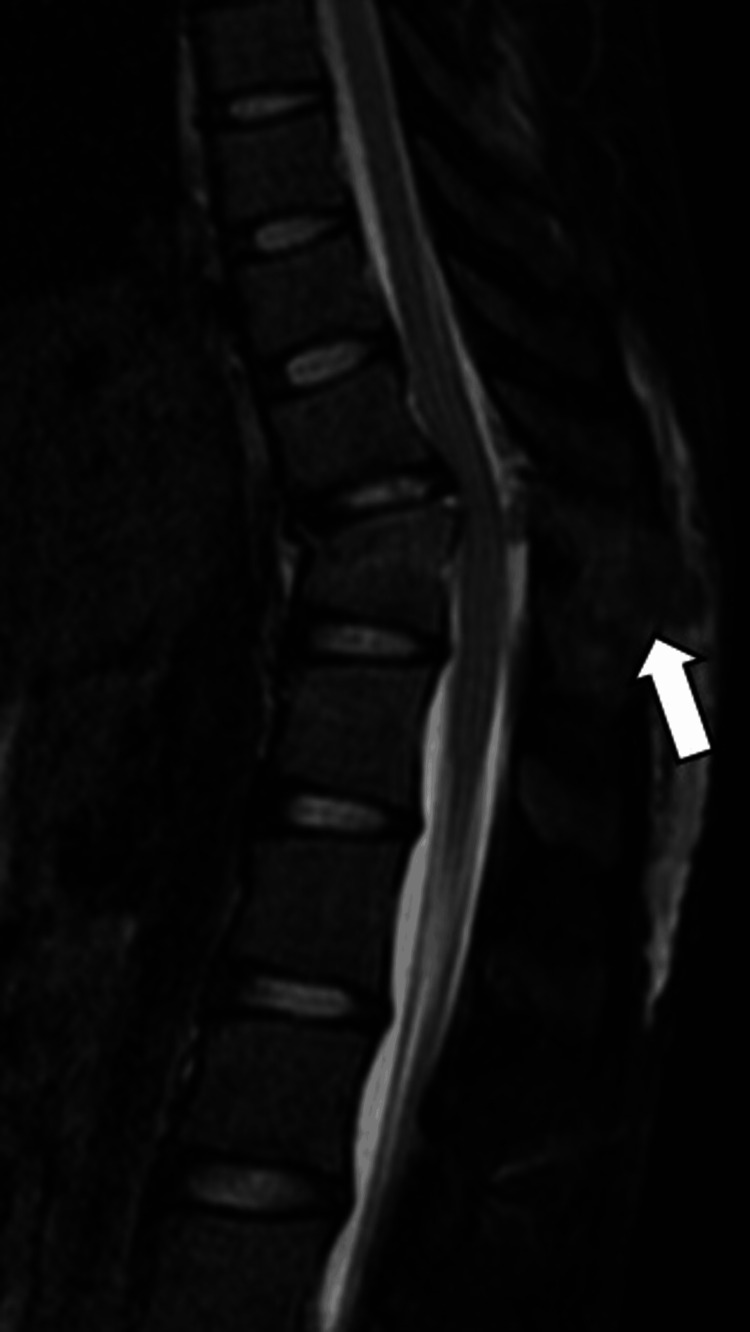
Preoperative plain thoracic magnetic resonance imaging Preoperative plain thoracic magnetic resonance imaging showing no signal intensity changes in the spinal cord, with evidence of posterior ligamentous complex injury (white arrow). There was a compression wedge fracture of T11.
